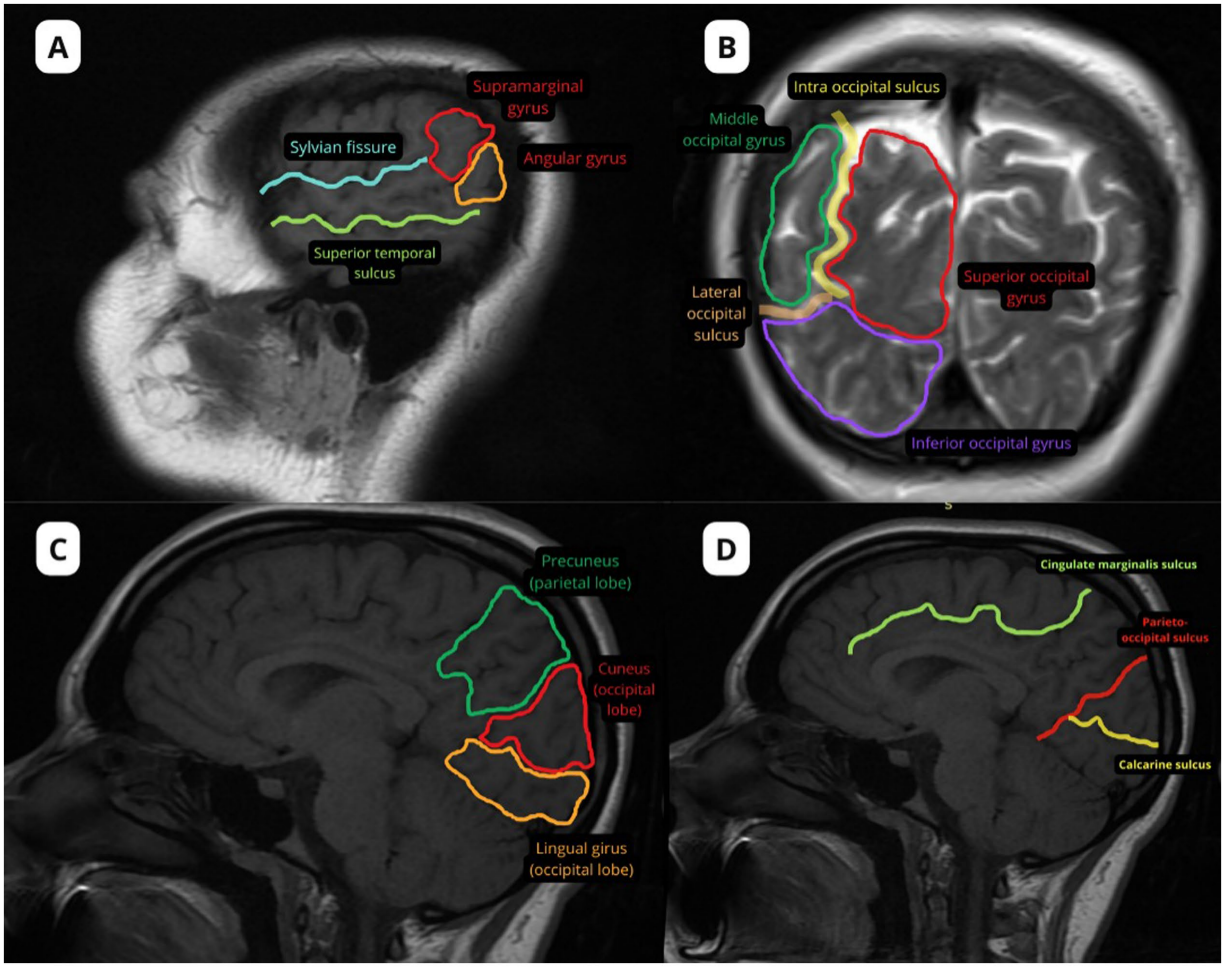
Main anatomical landmarks in the posterior region. Images were acquired using a GE scanner with a magnetic field strength of 1.5 Tesla. A pre-contrast T1-weighted sequence was used to highlight the alterations in A,C,D, while a T2-weighted sequence was used to highlight the alteration in B. (A) Supramarginal gyrus and angular gyrus; (B) Intra-occipital sulcus separating the superior occipital gyrus from the middle occipital gyrus, and lateral occipital sulcus separating the middle occipital gyrus from the inferior occipital gyrus; Precuneus (parietal lobe); cuneus and lingual gyrus (occipital lobe); (D) Marginal sulcus of the cingulate anterior to the precuneus, parieto-occipital sulcus separating the parietal lobe from the occipital lobe, and calcarine sulcus separating the cuneus from the lingual gyrus.
